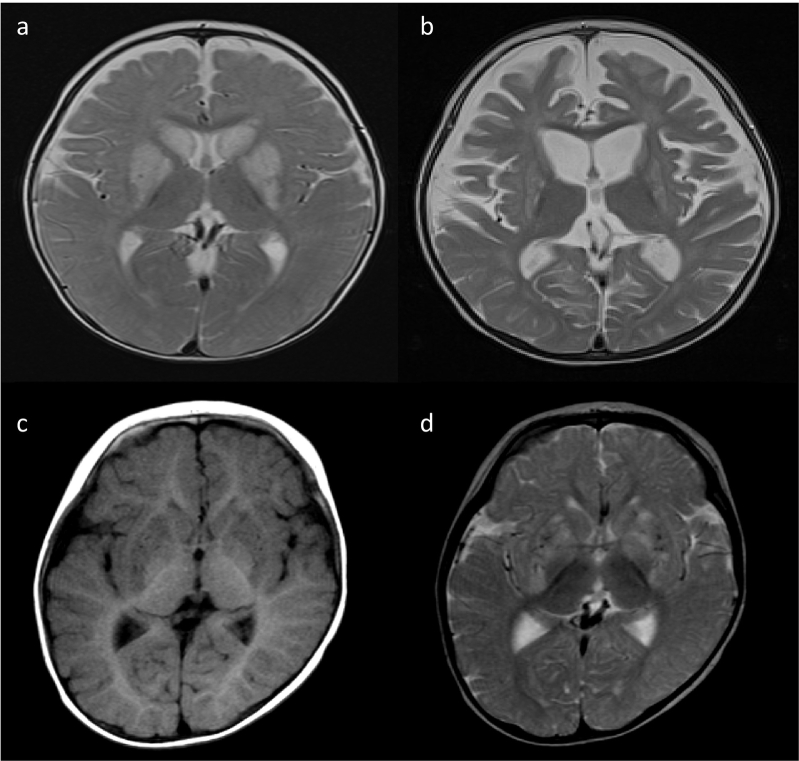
MRI findings. High intensity is observed in the striatum and globus pallidus on T2-weighted images (T2WI) at 8 months of age: (a) atrophy of the cerebral cortex and basal ganglia is observed on T2WI at 19 months (b) in patient 1. Low intensity is observed in the putamen, globus pallidus, and caudate nucleus on T1-weighted images (T1WI) at 10 months of age (c) and high intensity is observed in the putamen, globus pallidus, and caudate nucleus on T2WI at 10 months of age (d) in patient 2.
